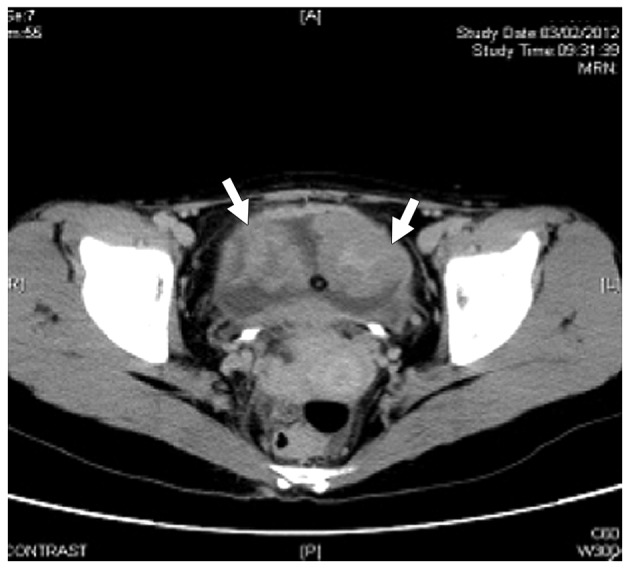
Computed tomography (CT) scan of the abdomen. Arrows indicate urinary bladder masses.
